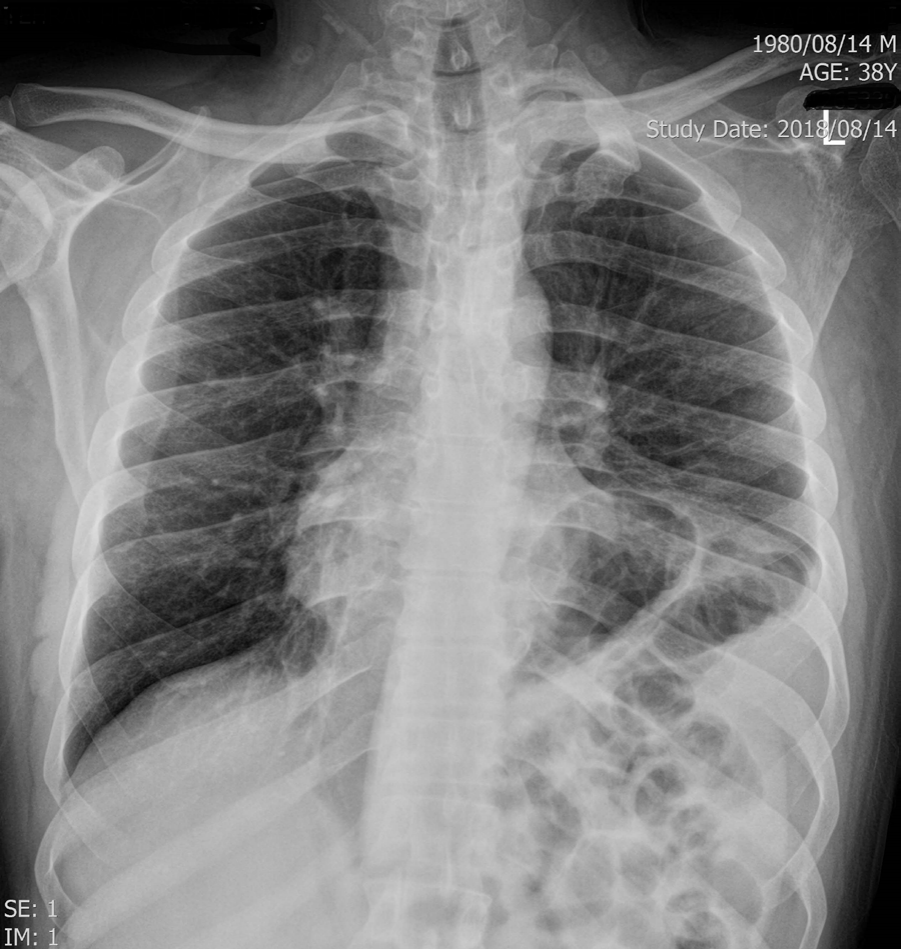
Chest X-ray in the posterior-anterior view, showing that the cardiac apex is not on the left or the right
